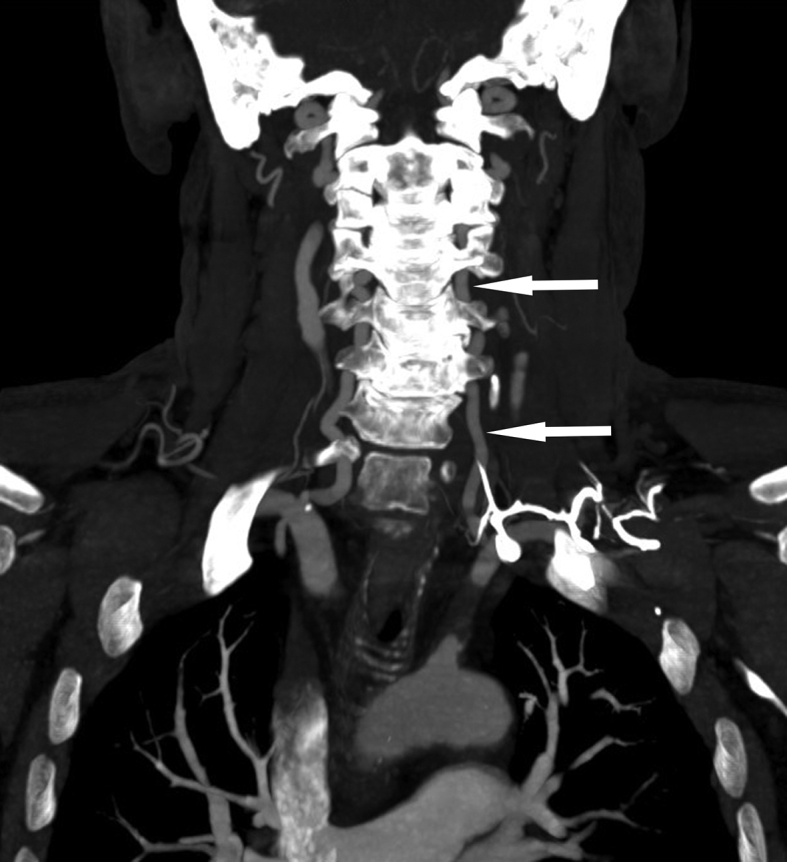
Contrast-enhanced CT angiography of the arterial neck vessels, coronal section. This image better demonstrates the flow gap within the proximal left subclavian artery and again demonstrates normal contrast filling of the left vertebral artery (arrows).
